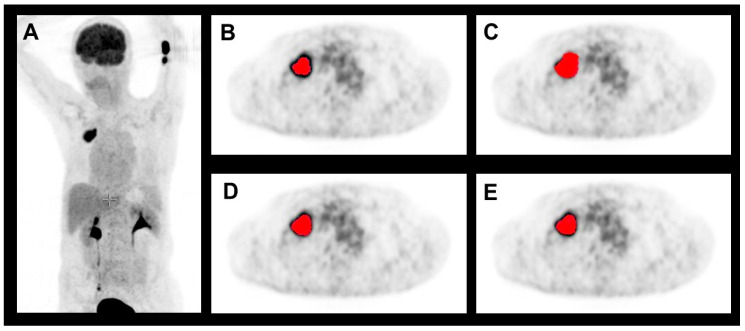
Example of a segmented lung lesion (in red) in FDG-PET for the four different segmentation algorithms with (A) showing the maximal intension projection of the patient. The pathological volume was measured as 38.8 ccm, which was most closely matched by the LE-based segmentation with 41.0 ccm (D) and the LZE-based segmentation with 39.1 ccm (E). As was the case for average values, in this case the threshold-based segmentation showed a volume of 31.1 ccm (B) which was smaller than the pathological volume, while the r-based segmentations showed a larger volume. The volume obtained using the KU-based segmentation was largest, at 49.2 ccm (C).
